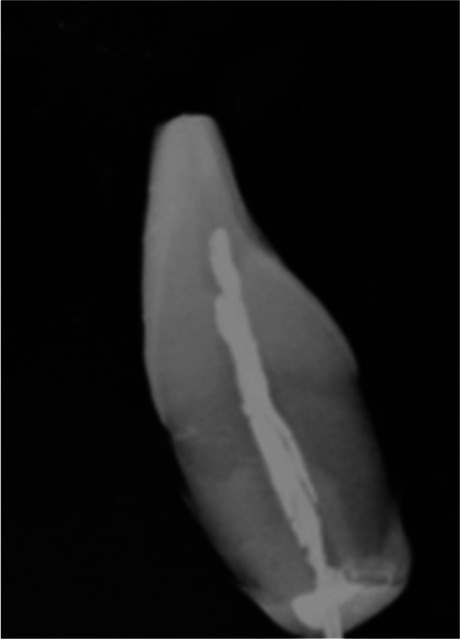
The detection of the exact location of the thermocouple inside the pulp chamber using radiographic images
